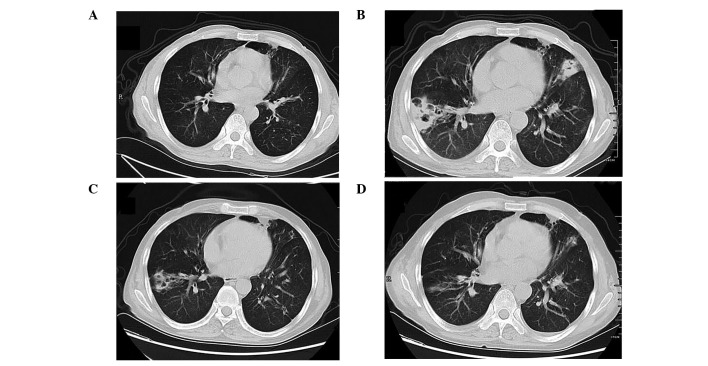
A chest computed tomography (CT) scan of a 47-year-old male with allergic pulmonary aspergillosis and pulmonary nocardiosis. Seven weeks prior to the onset of nocardiosis (A: 21/1/2013), the lung window image shows no notable lesions. On day eight following the onset of illness (B: 21/3/2013), the lung window image shows multiple new nodules with cavitation in both lungs. CT scans (C: 16/4/2013 and D: 28/5/2013) demonstrated clear improvements in inflammation following antibiotic therapy.
